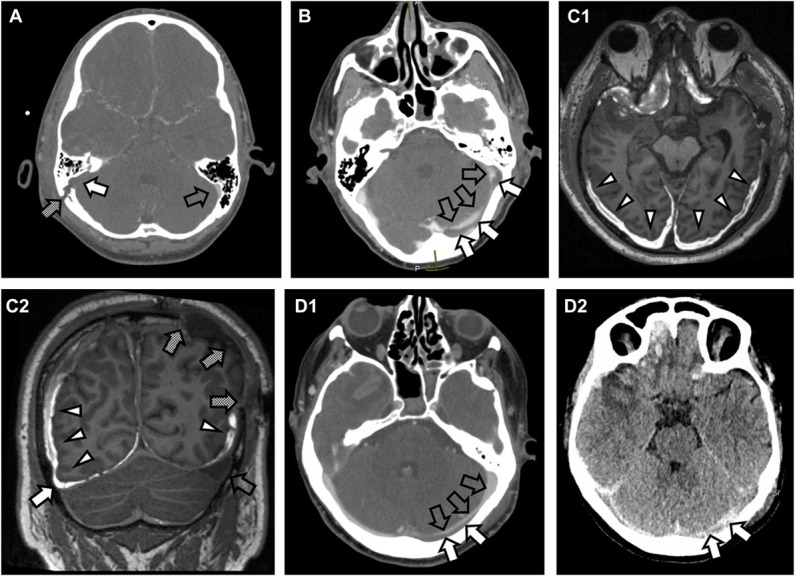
Representative scans of different intracranial pathologies causing post-traumatic VOC. A, A CTV showing absence of contrast in the right sigmoid sinus (white arrow) adjacent to a skull fracture (shaded arrow), suggestive of an occlusive sinus thrombosis. Contrast is present in the left sigmoid sinus (transparent arrow). B, A CTV of incomplete contrast enhancement of the left transverse and sigmoid sinuses (transparent arrows) associated with a low-density filling defect in the transverse sinus (white arrows) associated with a skull fracture crossing the sinus, suggestive of a nonocclusive sinus thrombosis. C1, A semiaxial gadolinium contrast-enhanced T1-weighted magnetic resonance scan showing subdural hematomas (white arrowheads). C2, The subdural hematomas cause narrowing of the nondominant right transverse sinus (white arrow), suggestive of extrinsic compression of the sinus. The dominant left transverse sinus (transparent arrow) has expanded because of an ipsilateral decompressive craniectomy (shaded arrows) and the right-sided VOC. D1, A CTV of narrowed contrast-enhancement of the left transverse sinus (transparent arrows) because of a thin skull fracture–associated epidural collection (white arrows) displacing the sinus away from the inner table of the skull without fully occluding the sinus. D2, The epidural collection (white arrows) is better visualized in the non–contrast-enhanced CT scan. CT, computed tomography; CTV, CT venography; VOC, venous outflow compromise.
